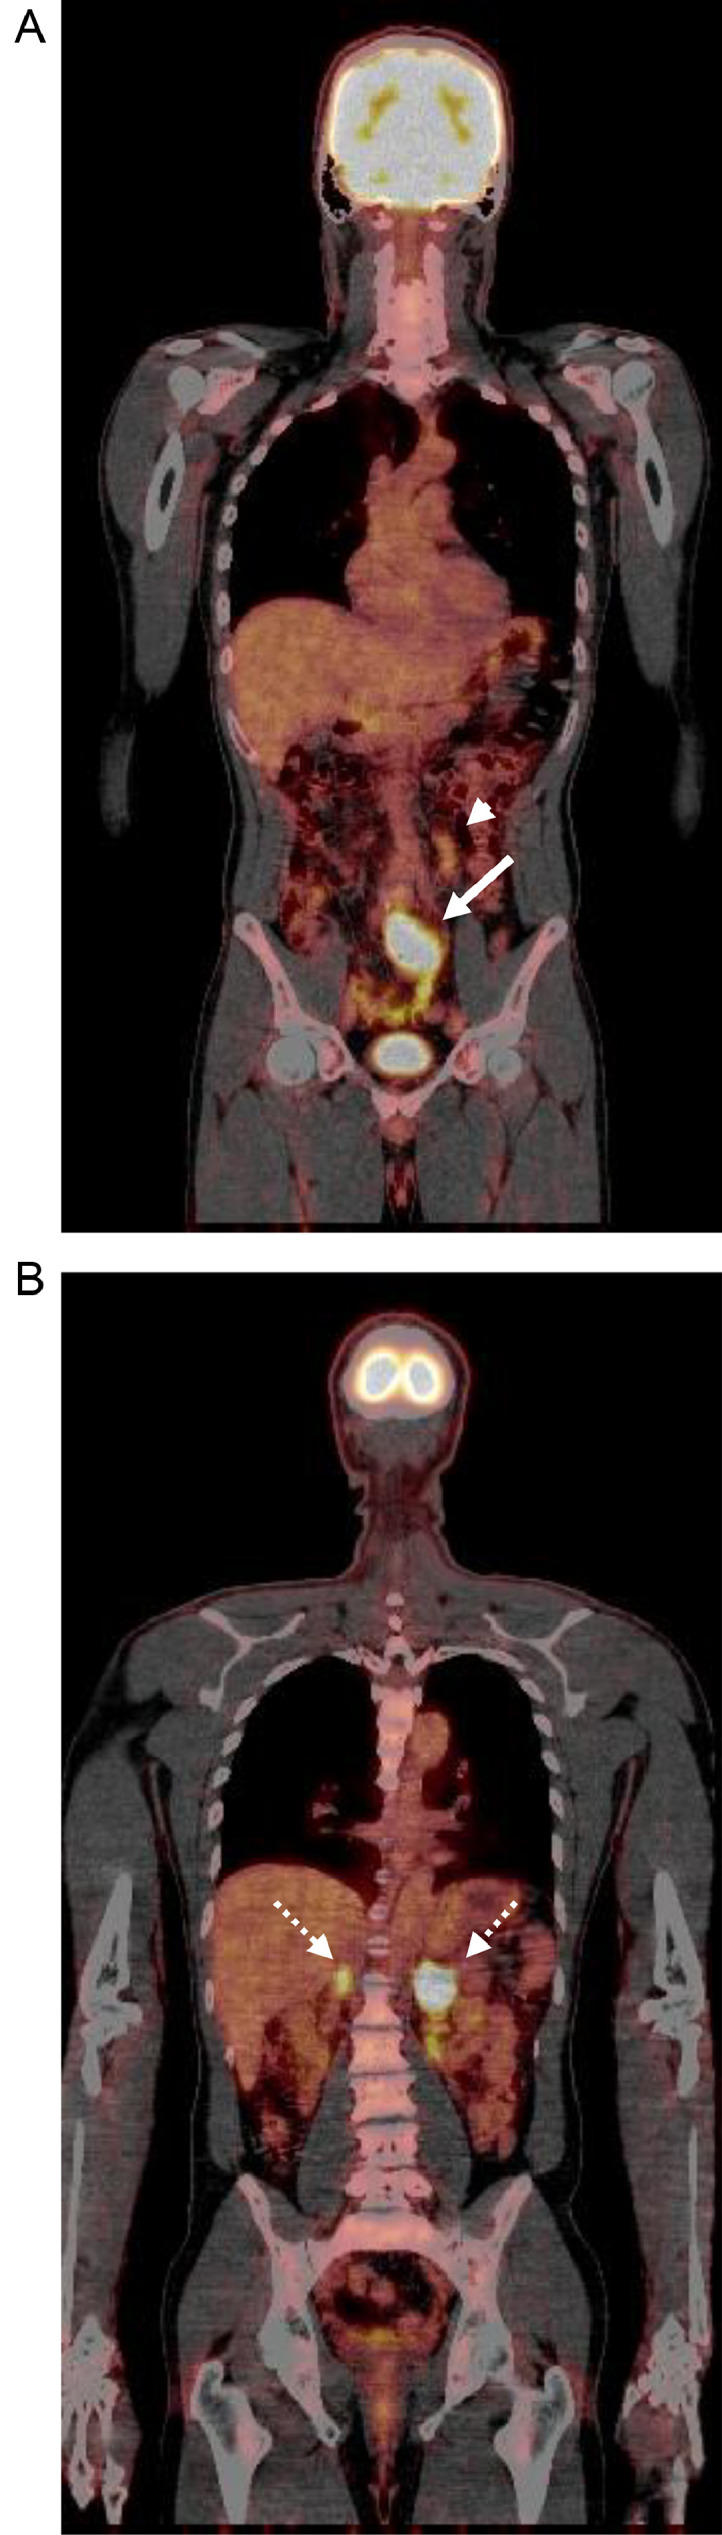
PET-CT images. Coronal PET-CT images show FDG uptake in the jejunal lesion (A: white arrow), a lymph node (A: arrowhead) and bilateral adrenal lesions (B: dotted arrows). SUV max values: jejunum = 10.3; lymph node = 3.3; left adrenal = 21.8; right adrenal = 15.
